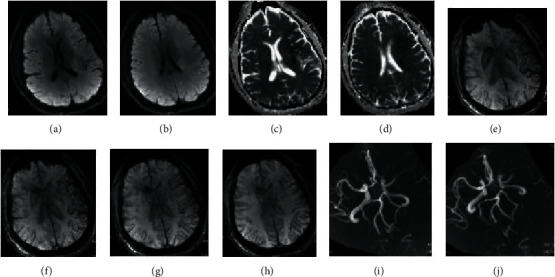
MRI image of a patient. Note: the patient was a 49-year-old man with impaired right limb movement and slurred speech for 2 hours. In (a) and (b), DWI shows patchy higher signal intensity in the left frontal lobe, insula, and lateral ventricular asides (hyperacute ischemic stroke). In (c) and (d), ADC suggests patchy low signal in the voiceover area of the left frontal lobe, insula, and lateral ventricle. In (e)–(h), SWAN indicates increased distribution of draining and decreased signal of left frontal temporal lobe and insular pia meningeal. In addition, the distribution of medullary veins in the voiceover area of the lateral ventricle increased and signal decreased (positive PVS). In (I) and (j), 3D-TOF MRA suggests occlusion of the M1 segment of the left middle cerebral artery.
